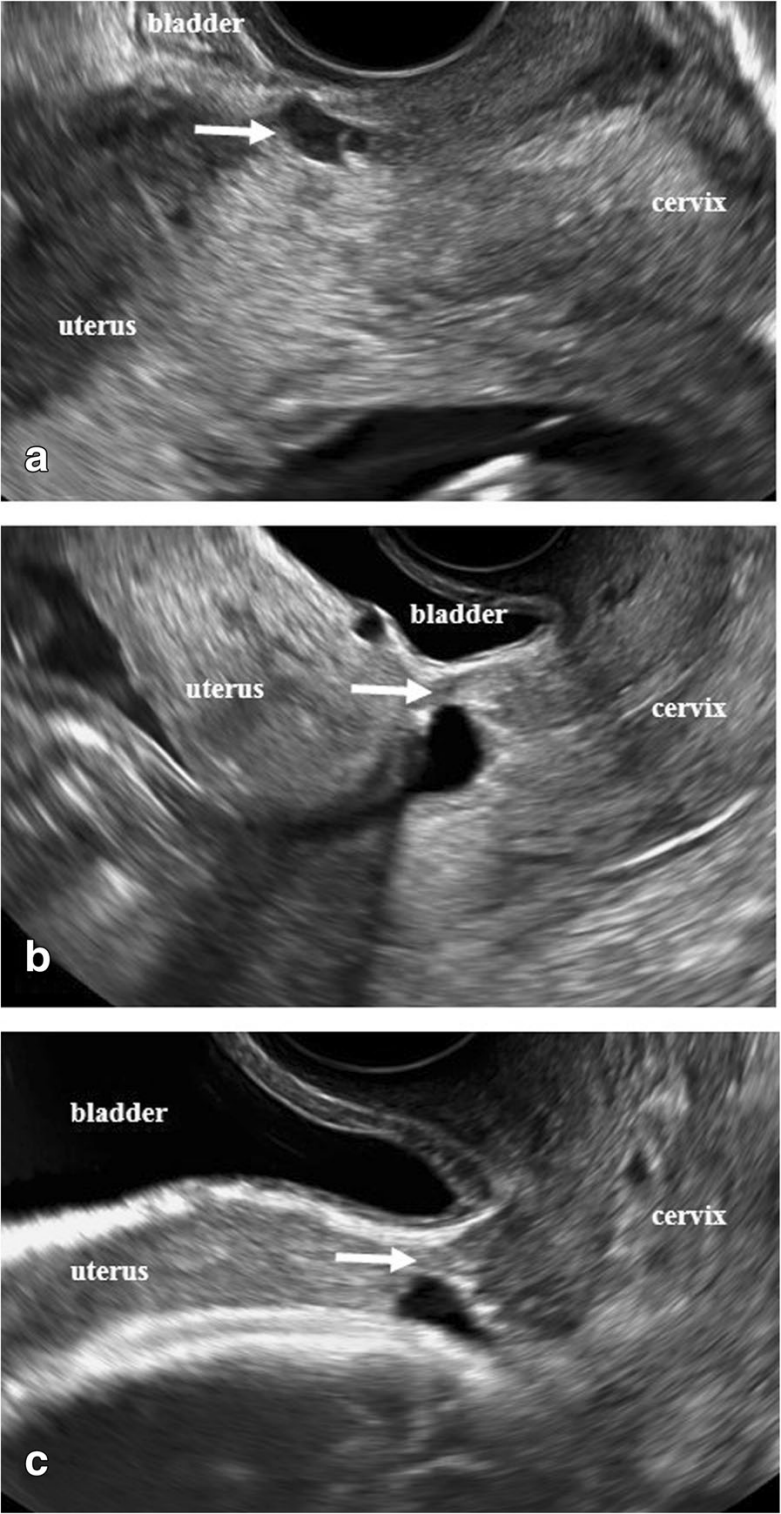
Transvaginal sonographic images. The *arrows* indicate the appearance of the cesarean scar area at the presence of the scar pregnancy at 13 + 2 (a) and after reabsorption of the scar pregnancy at 22 + 0 (b) and at 30 + 2 (c) weeks of gestation
